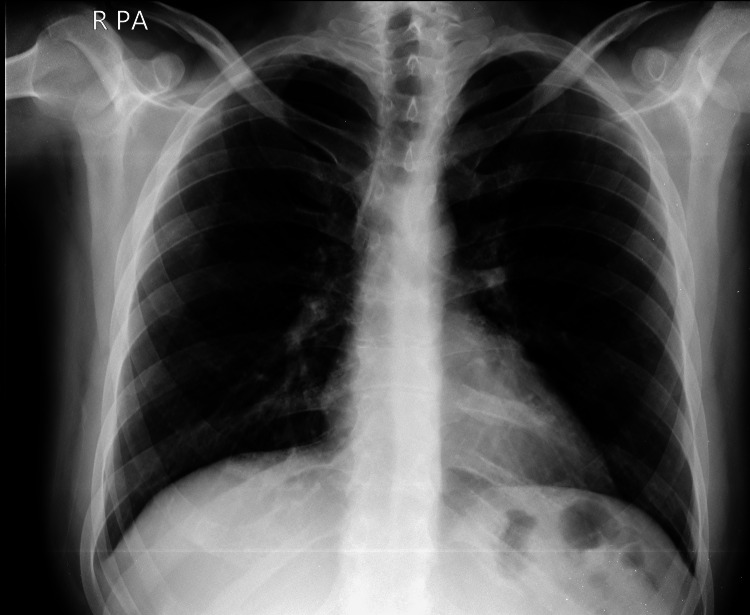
A 32-year-old male with a chest radiograph affected by X-ray overpenetration.
